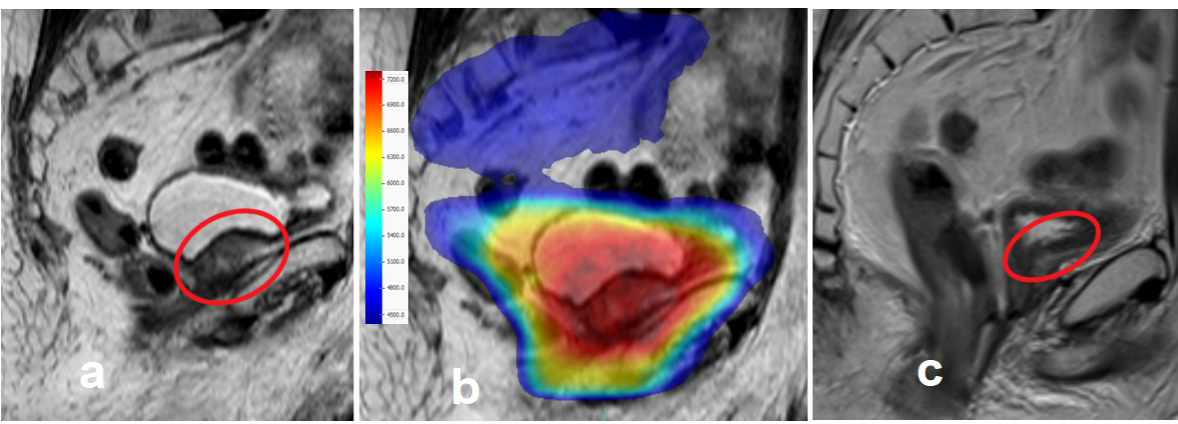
An exemplary case treated with MRI-guided prostate cancer radiotherapy. (A) Macroscopic prostate bed relapse near the intestines 15 years after radical prostatectomy. (B) The sum of dose distributions to the pelvic lymph nodes, prostate bed, and relapse area. (C) Total macroscopic regression of the tumor, 3 months after radiotherapy.
